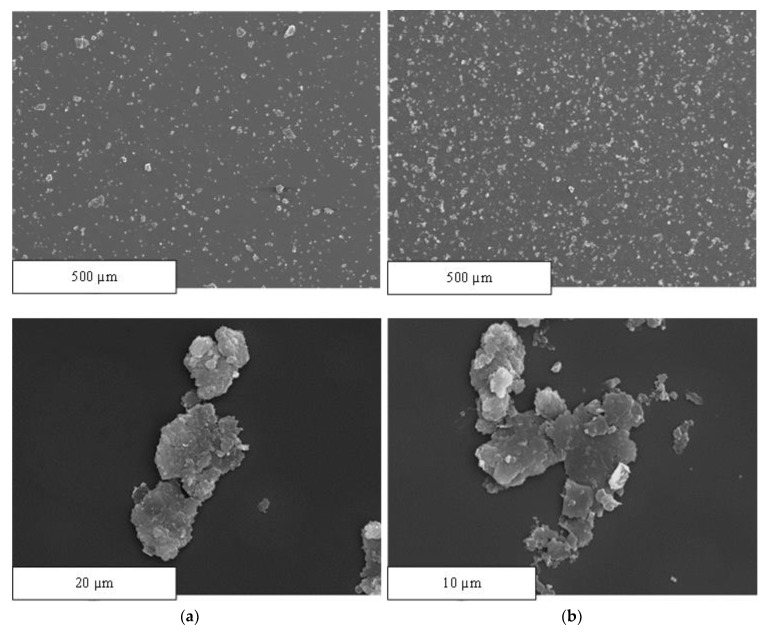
SEM images of carbon nanostructure samples: (a) sample prepared without ultrasound (×100 and ×2500), (b) sample prepared with an ultrasonic bath after manual mixing (×100 and ×4500).
